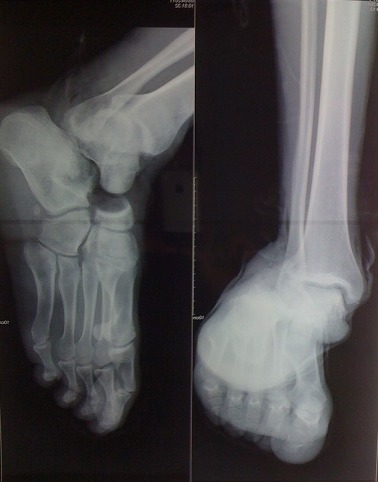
Radiographie standard de face et de profil de la cheville gauche montant une luxation sous-talienne externe
